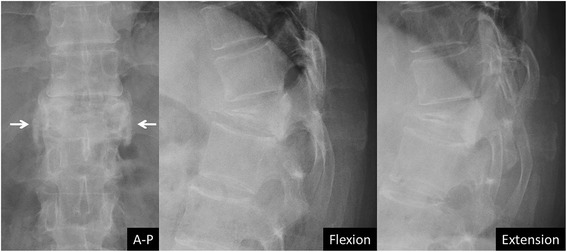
Bridging bone around a vertebral cleft. Although a vertebral cleft was observed, the presence of bony bridging around the fractured vertebra (arrows) was interpreted as diagnostic of radiographic union
